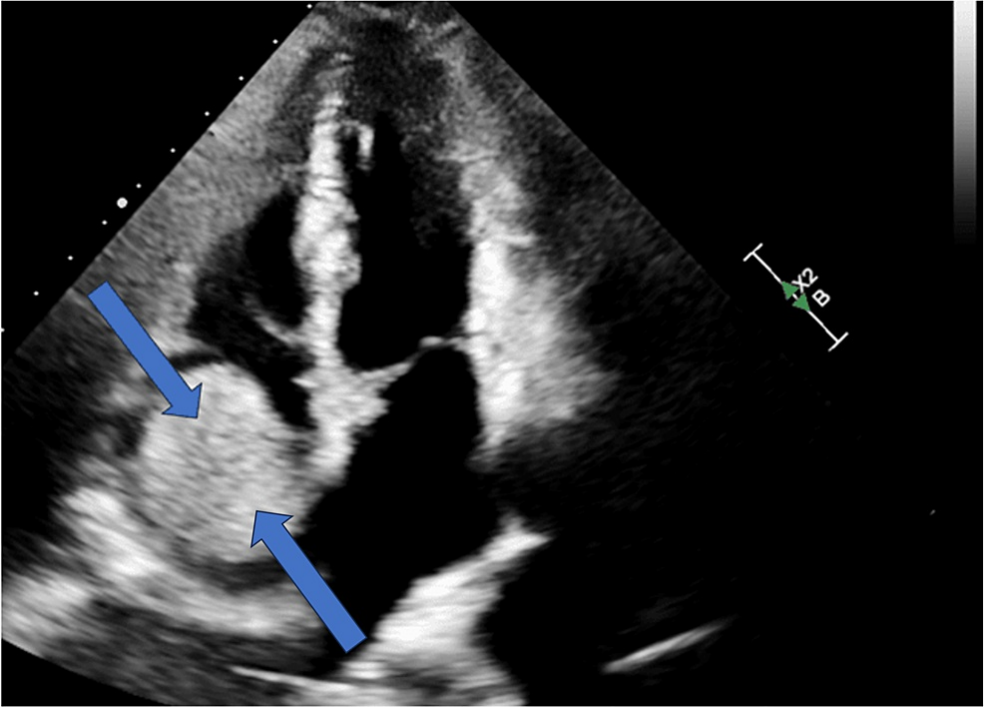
Apical four-chamber view of TTE again depicting a large mass in the right atrium (blue arrows) TTE: transthoracic echocardiogram
